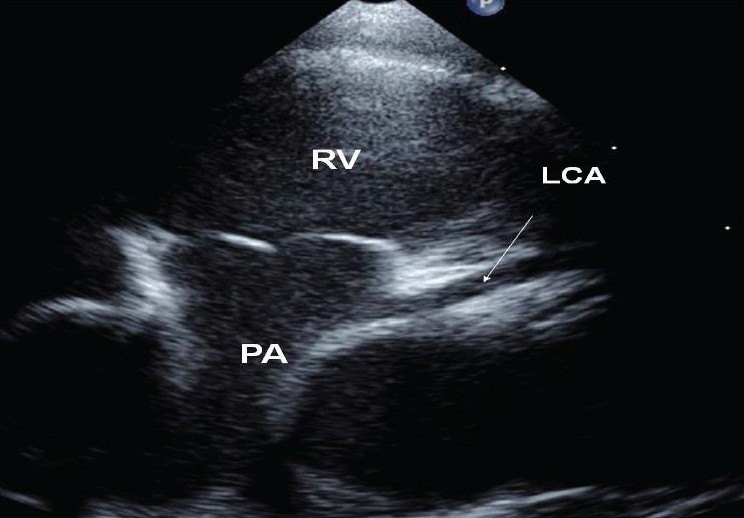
Modified parasternal view showing origin of left coronary artery from pulmonary artery. LCA: left coronary artery, PA: pulmonary artery, RV: right ventricle.
